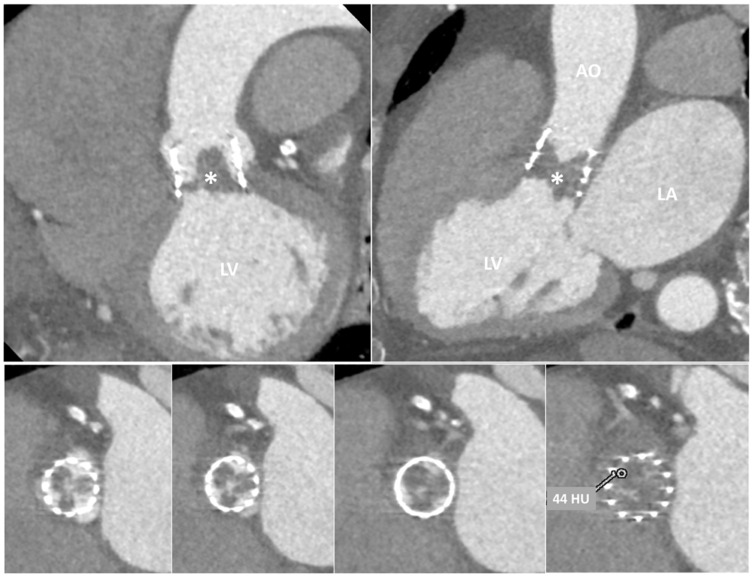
Contrast CT in a patient with transcatheter aortic valve. Upper panels: oblique views of the prosthetic valve (PV); the asterisk indicates the presence of a hypodense mass adherent to prosthetic leaflets. Lower panels: transverse planes of PV showing complete obstruction of the valve; the HU at the level of the mass was 44, indicating the thrombotic nature of the mass. AO, aorta; LA, left atrium; LV, left ventricle.
